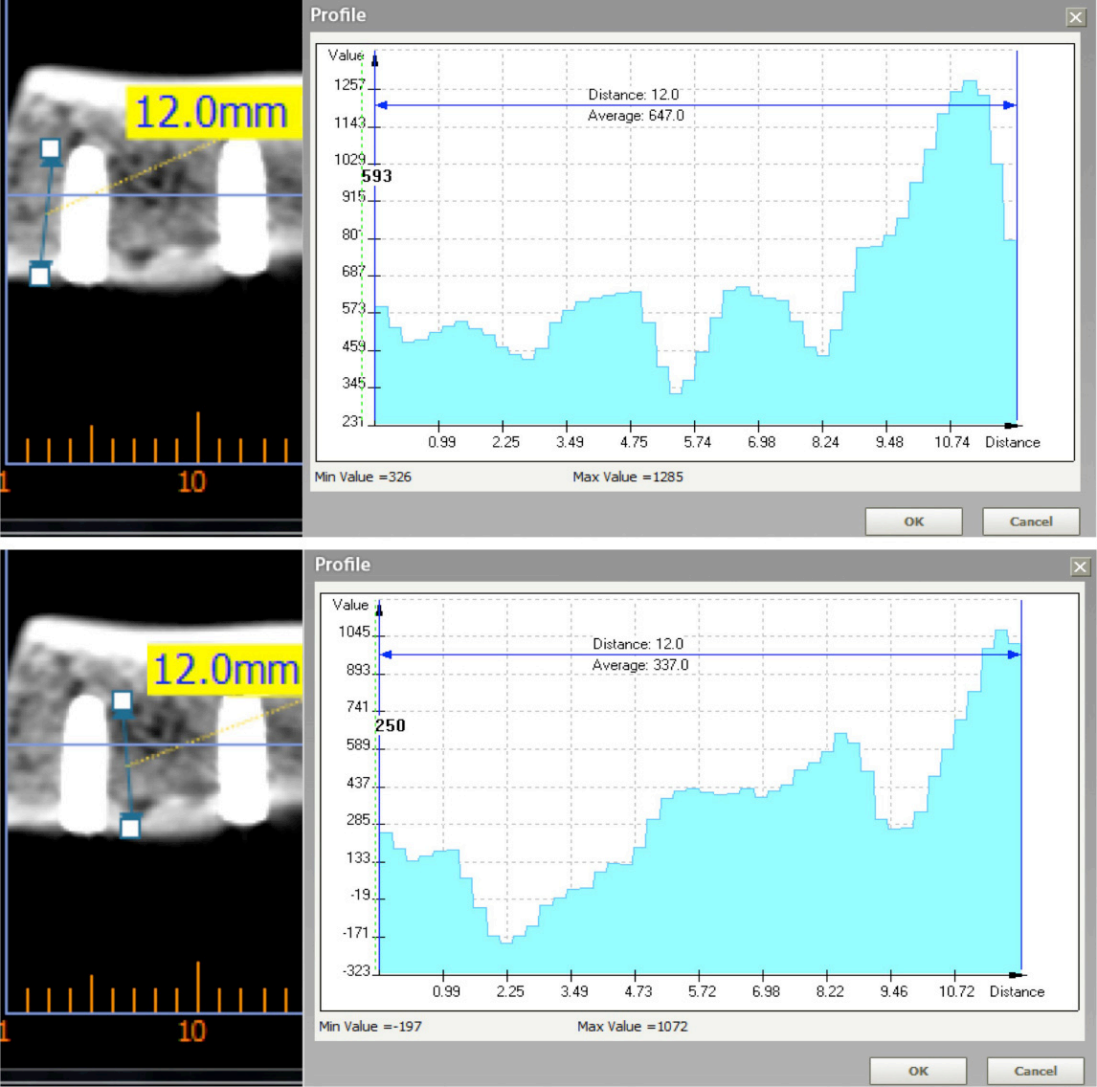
Panoramic section of a CT scan. Bone quality average recorded in HU of the implant recipient area measured on each side of it using Ez3D plus software.
